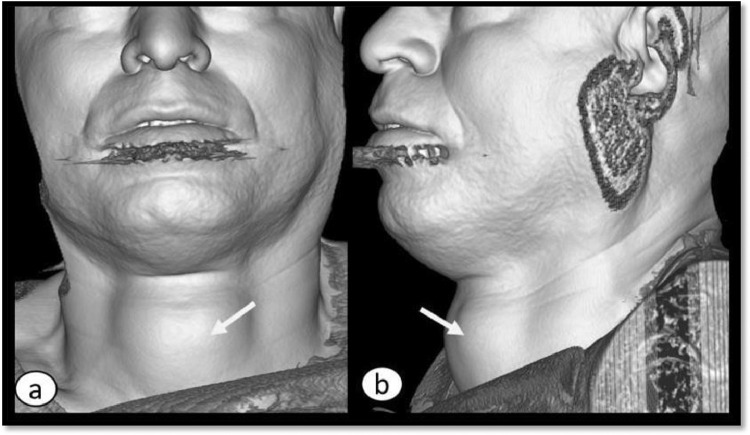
(a,b): 3D CT volume rendered images for skin, showing midline neck swelling (arrows).
